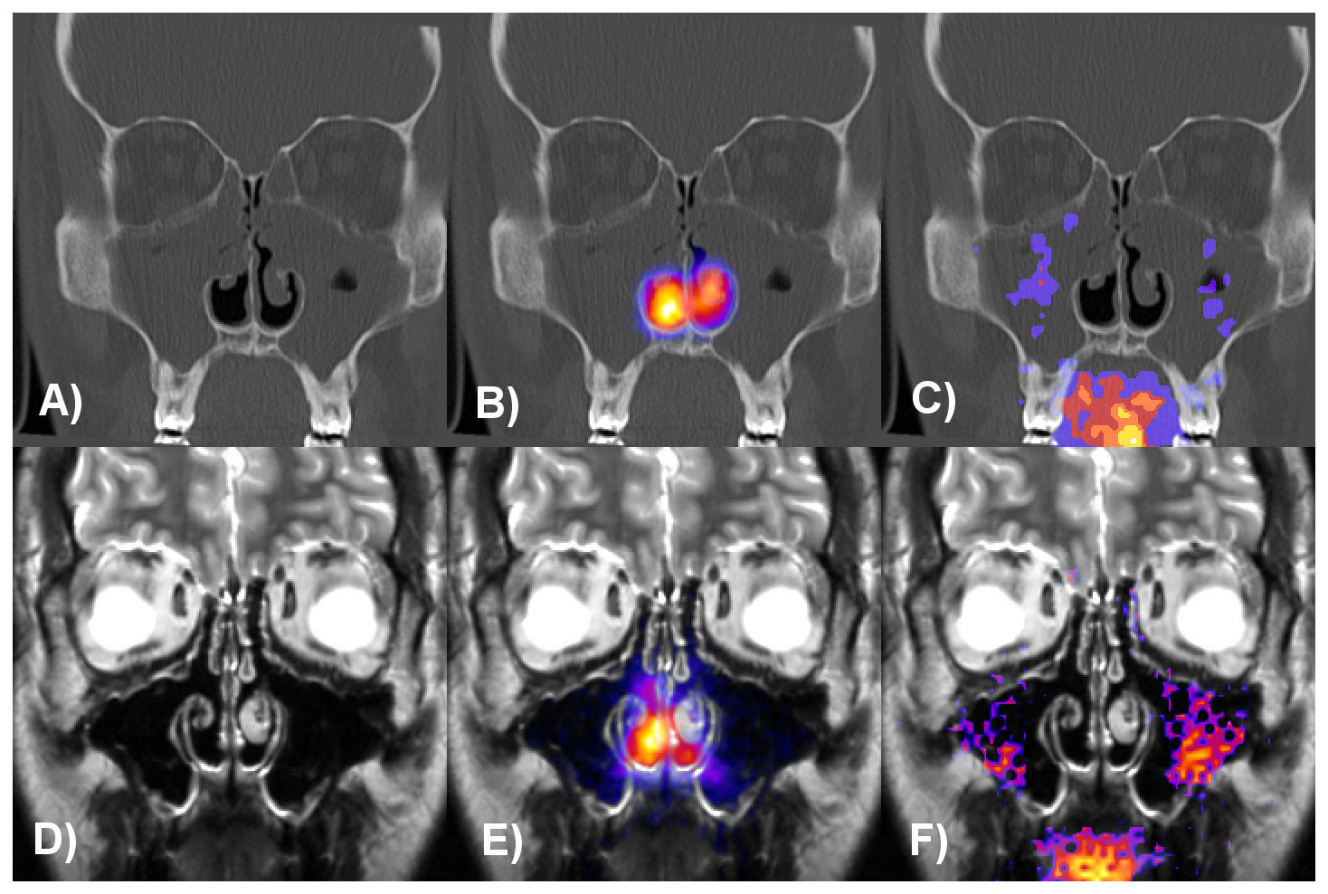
Coronal CT and MRI slices of a CRS patient before and 166 days after sinus surgery. Superposition of the anterior gamma camera images with the CT slice before FESS (A–C) without (B) and with (C) central nasal lead shield mask and with the MRI slice after FESS (D–F) without (E) and with (F) lead shield. Lund-Mackay score was 15 and maxillary sinus deposition was 1.3% (C) before and 7.9% (F) after surgery. The patient had septoplasty and conchotomy on both sides eight years before participation in the study.
